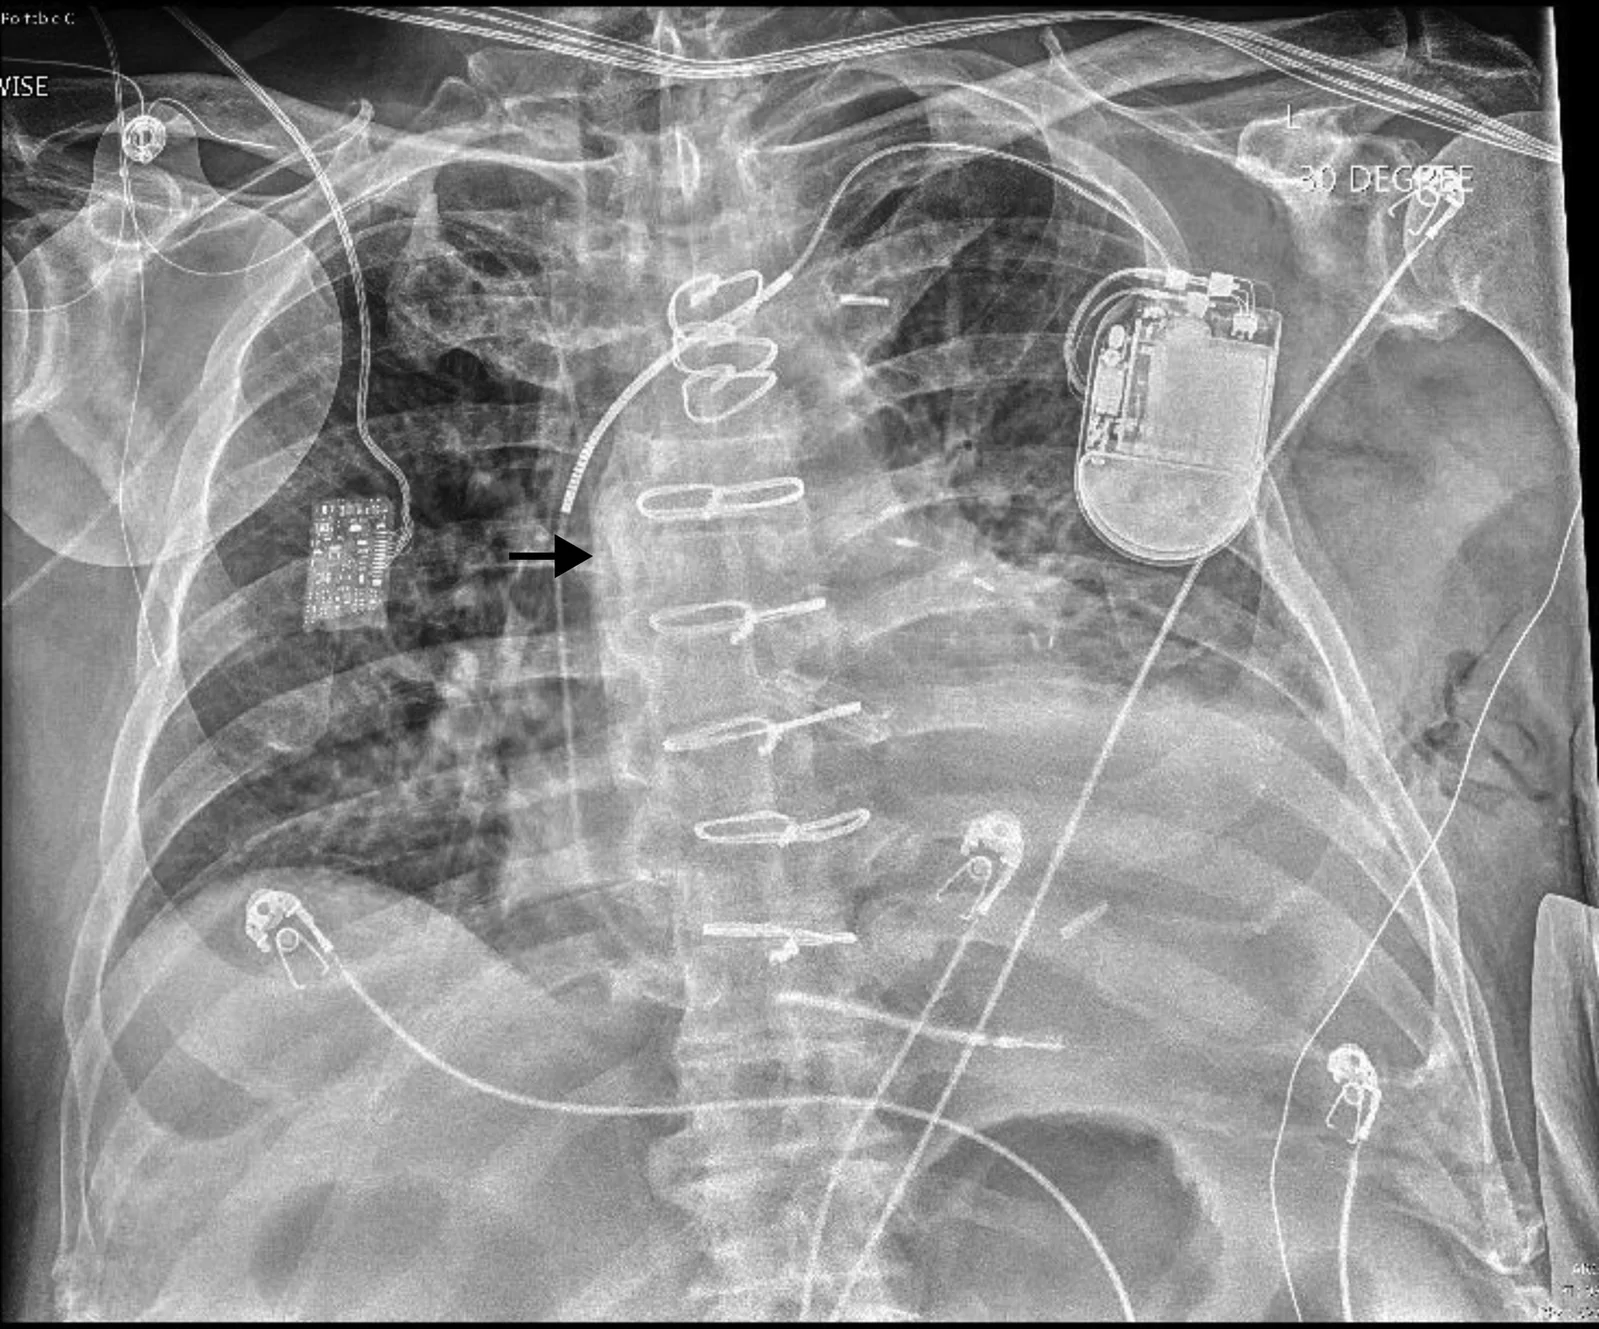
Anterior–posterior chest radiograph taken on postoperative day 7 showing the peripherally inserted central catheter tip in the lower third of the superior vena cava at the cavoatrial junction (black arrow).
